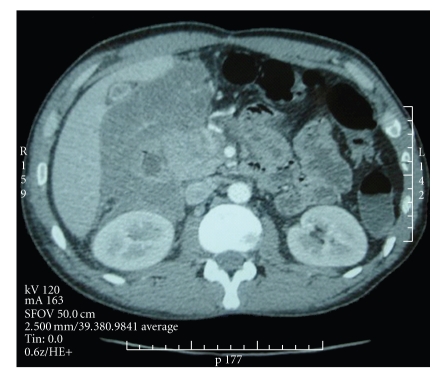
CT-scan showing the retroperitoneal mass.
